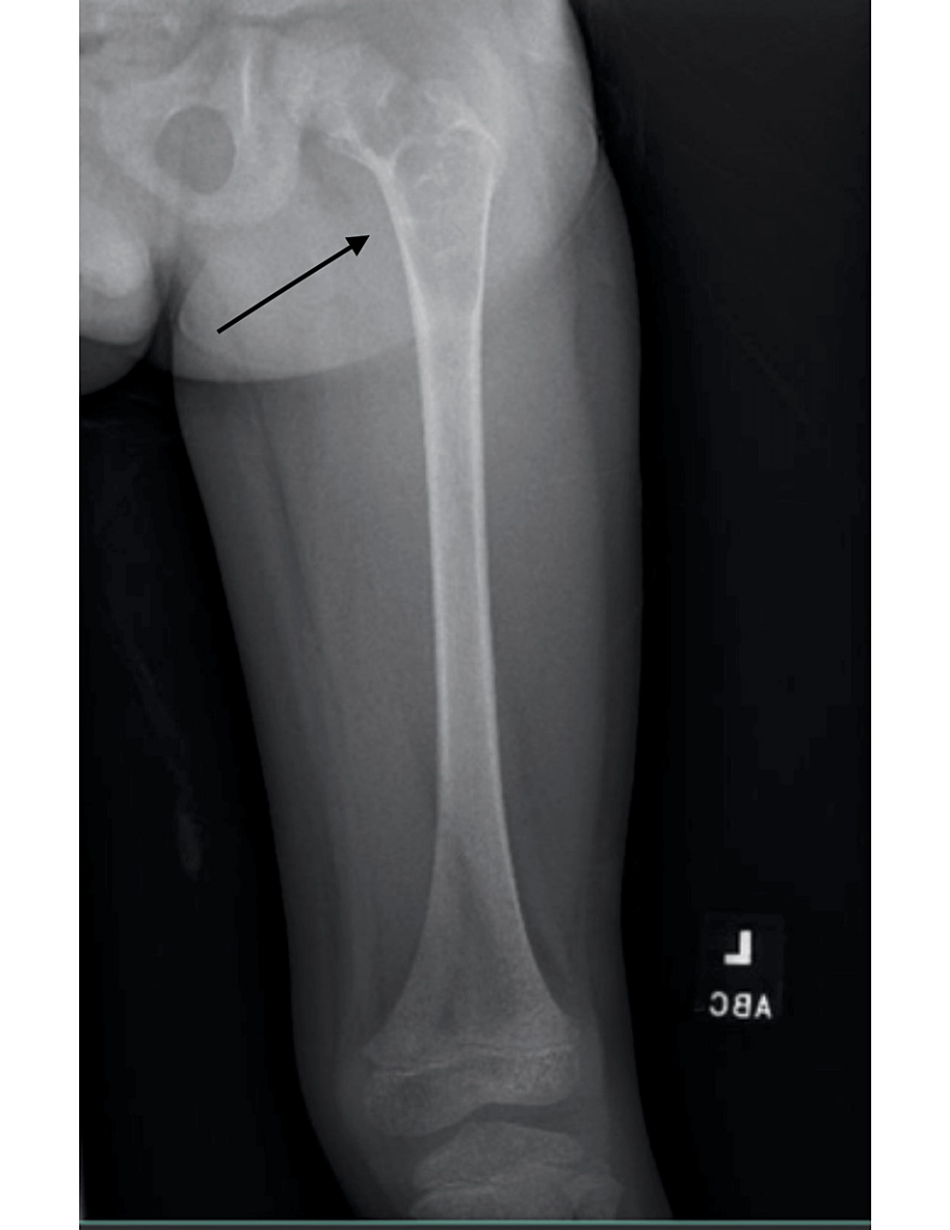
Radiograph showing an ill-defined lytic lesion of the left proximal femur with concern for pathologic fracture at the location of the arrow
